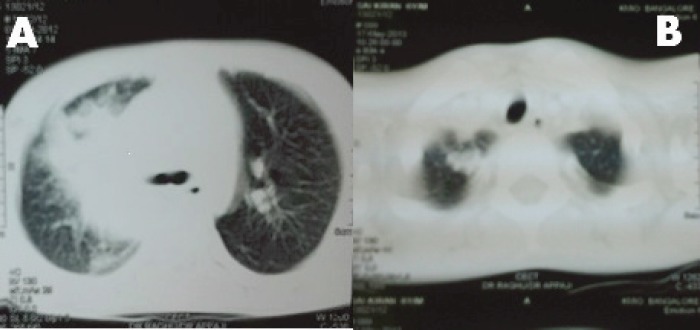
Panel A: computed tomography of thorax shows a soft tissue density lesion in the right lung measuring with a pleural-based nodule in the apical basal segment of right lower lobe. Panel B: computed tomography of thorax shows near total resolution of the soft tissue density lesion following chemotherapy and radiation.
